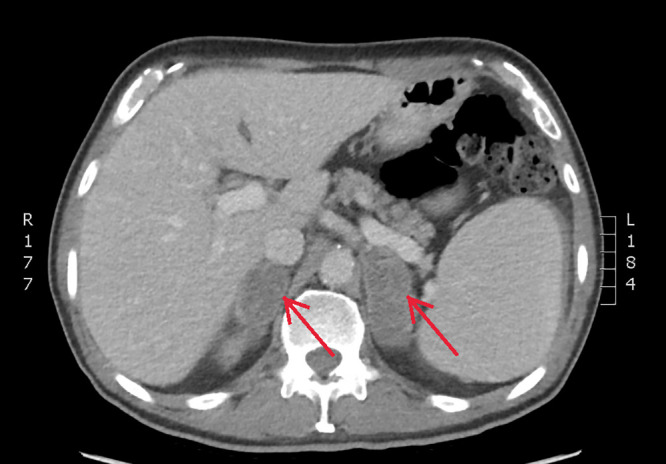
Computed tomographic (CT) scan of the abdomen, transverse view, showing bilateral adrenal masses (arrows) measuring 5.0 × 2.1 × 6.0 cm (anteroposterior [AP)], transverse [TV], craniocaudal [CC]) on the right and 6.1 × 2.6 × 5.7 cm (AP, TV, CC) on the left with heterogenous hypoattenuation. This figure appears in color at www.ajtmh.org.
